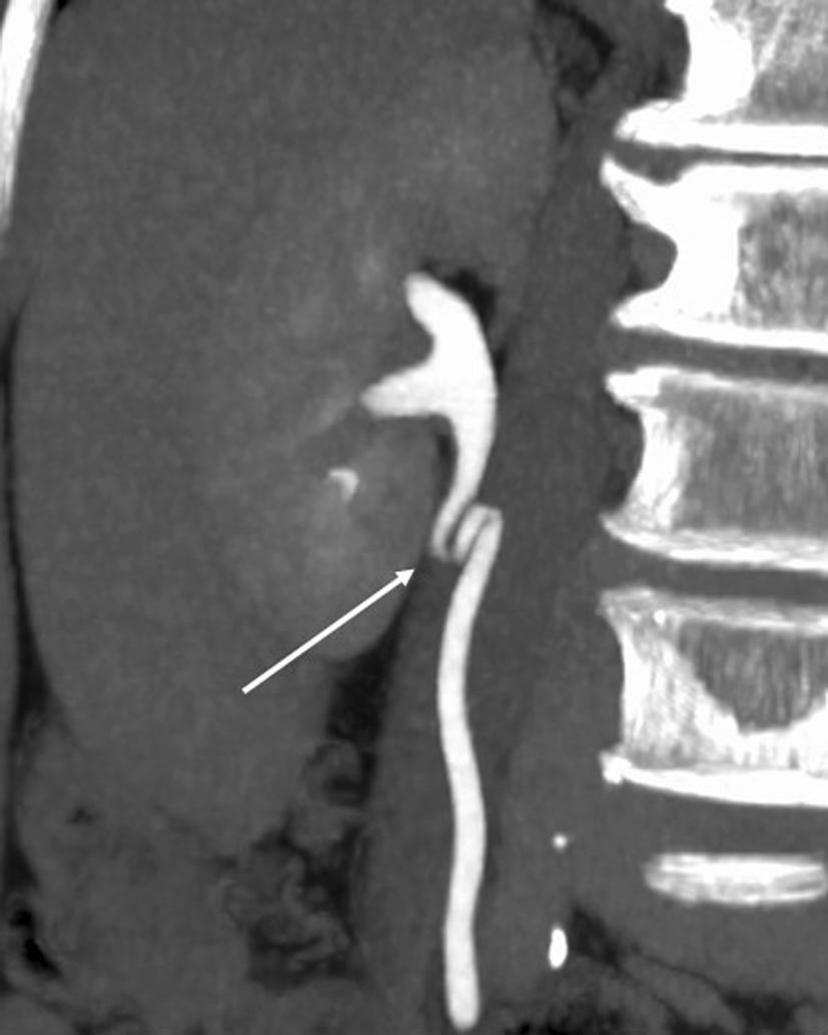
Kinking of the upper ureter in a reconstructed excretory coronal CT image of a 63-year-old male (cited from [12]). In retrograde pyelography or computed tomography (CT) urography, kinking of the upper ureter is not rare, although usually ignored
